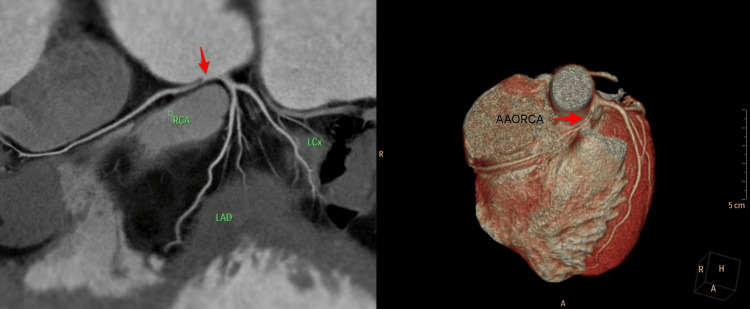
Preoperative computed tomography coronary angiography with reconstruction showing right coronary arising from left coronary cusp with short intramural course and long interarterial course. RCA: right coronary artery, LAD: left anterior descending, LCx: left circumflex, AAORCA: anomalous aortic origin of the right coronary artery
